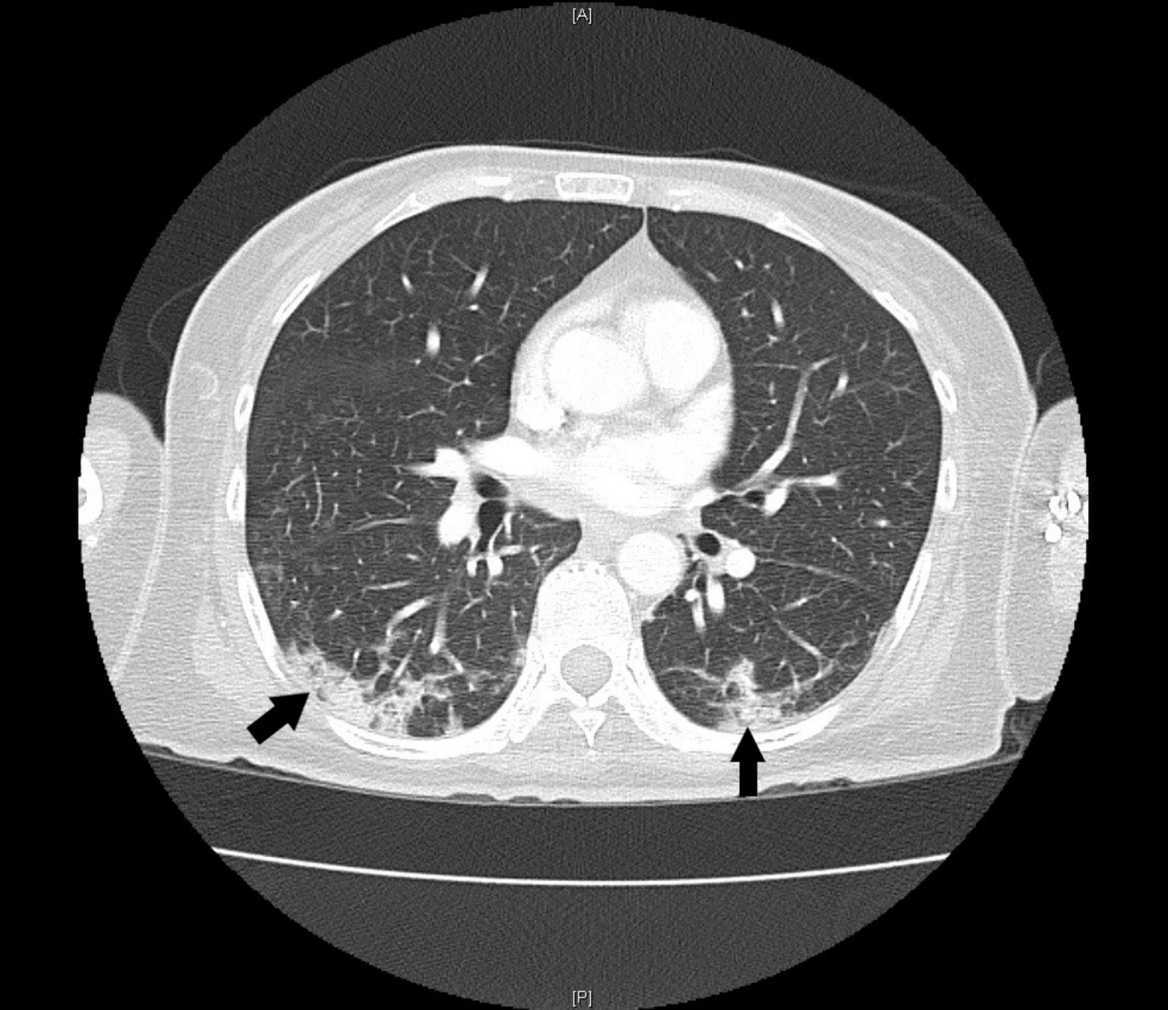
Computed tomography (CT) of the chest with intravenous contrast showing infiltrates in dependent portions of the lung (marked by arrows)
